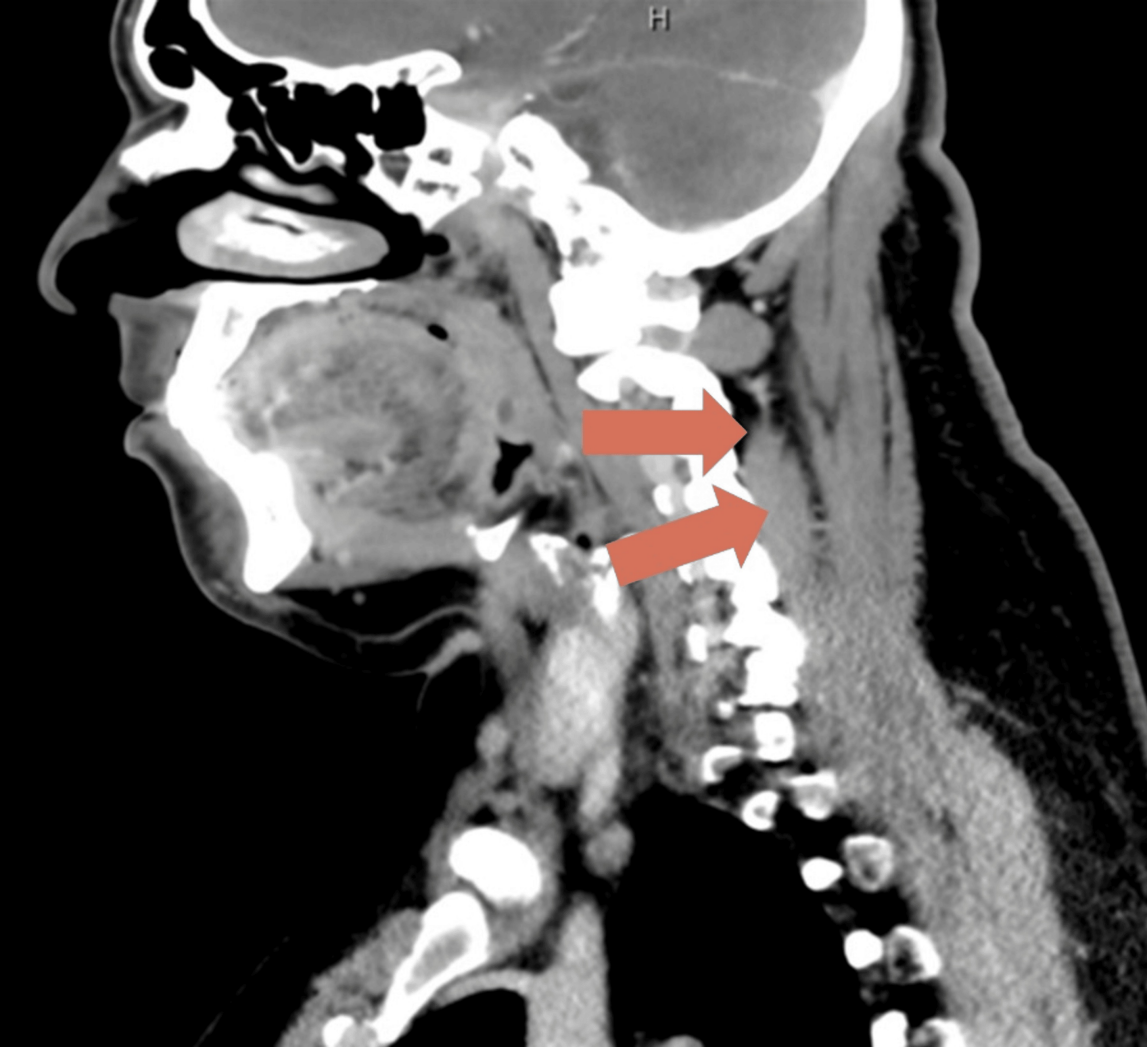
Complete resolution of cervical abscess on follow-up imaging Contrast-enhanced CT of the cervical spine obtained during outpatient follow-up showed complete resolution of the previously documented posterior cervical abscess. No residual fluid collections or soft tissue inflammation are seen.
